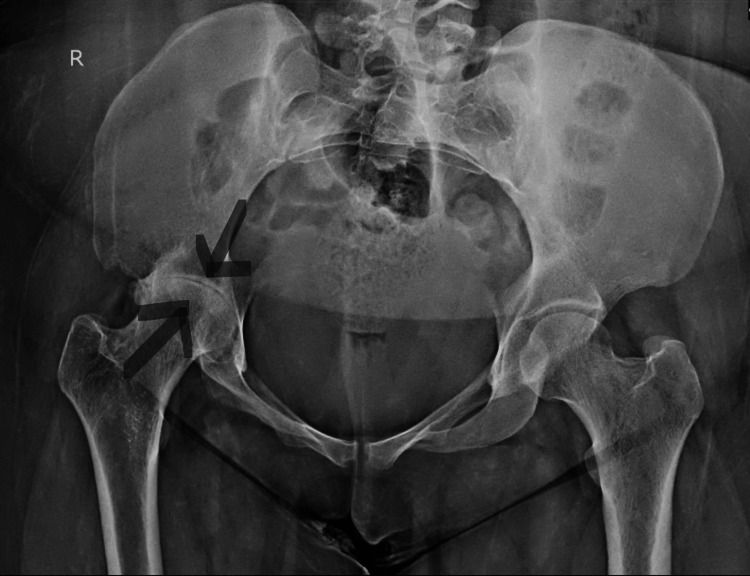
Preoperative X-ray Anteroposterior X-ray of the pelvis and hips indicates significant degenerative changes in the right hip joint, consistent with advanced osteoarthritis or chronic tuberculosis-related osteomyelitis. Findings include marked joint space narrowing, femoral head deformity, acetabular irregularity, sclerosis, erosion, and osteophyte formation.
